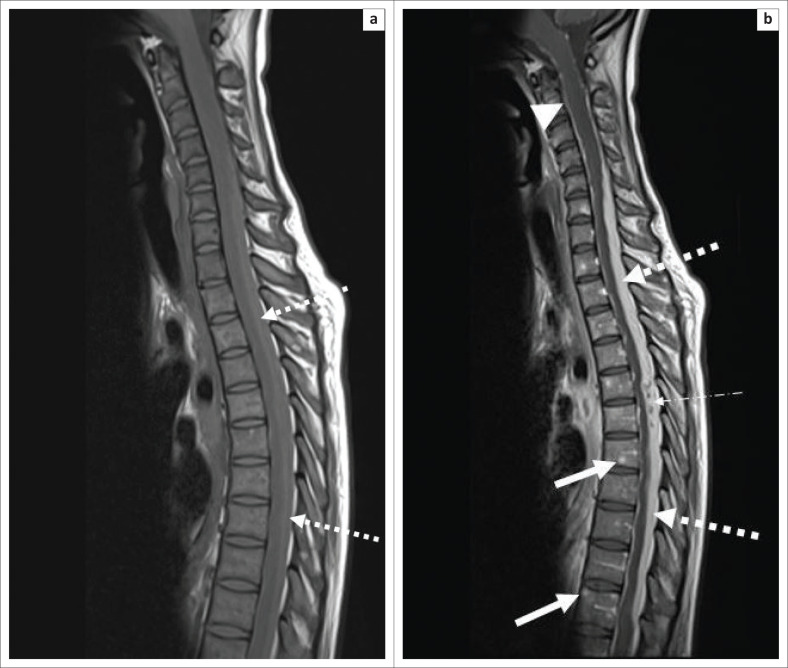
A 37-year-old female with advanced human immunodeficiency virus and disseminated tuberculosis presented with a 3-week history of bilateral lower limb weakness, urinary retention and loss of bowel control. (a) Sagittal T1-weighted pre-contrasted magnetic resonance imaging of the spine reveals inflammatory exudate along the posterior cord (white arrow with dashed line). (b) Sagittal T1-weighted post-contrasted magnetic resonance imaging of the spine reveals multiple enhancing microabscesses throughout the spine (solid white arrows), diffuse leptomeningeal enhancement along the brainstem and spinal cord (white arrow head) and long-segment enhancing inflammatory exudate along the posterior cord, which compresses the cord against the vertebral column (white arrows with dashed line) and contains central necrosis (white arrow with dotted dash line).
